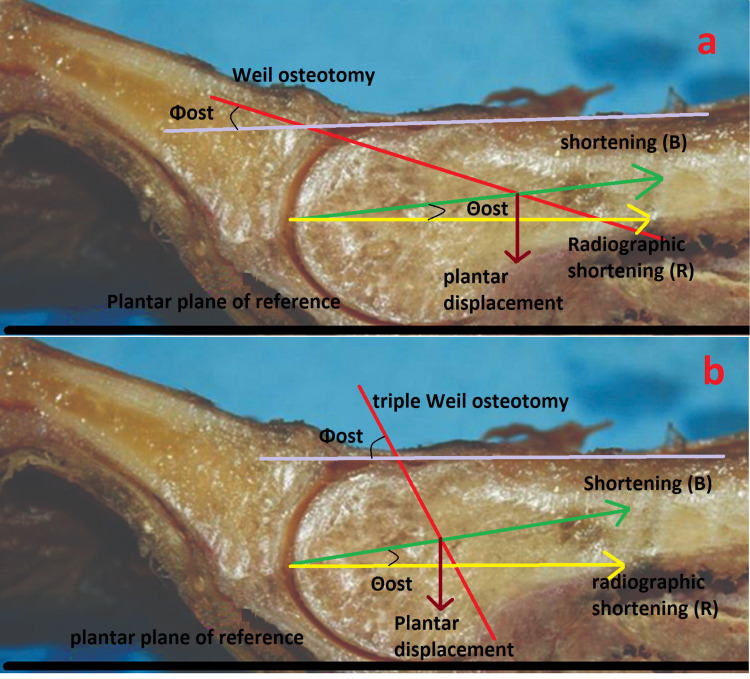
Weil osteotomy (A) and triple Weil osteotomy (B) The first panel shows a Weil osteotomy, where the proximal displacement of the head fragment is calculated using the most distal edge of the proximal fragment as a reference. The second panel shows triple Weil osteotomy, where the proximal displacement of the metatarsal head is measured by the thickness of the bone fragment removed by the second cut. In both cases, the shortening of the metatarsal (B) is along its longitudinal axis. The radiographic shortening (R) is along the plane of reference. Θost: osteotomy angle.
